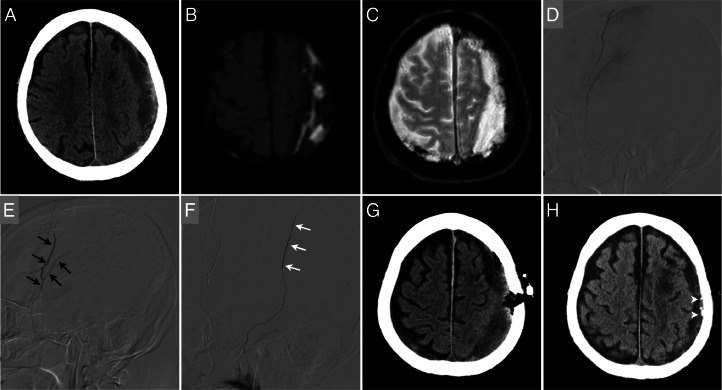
A 76-year-old male with right hemiplegia and thrombocytopenia was admitted. (A) A head computed tomography (CT) scan on admission showed a left subdural hematoma with a tough membrane and a slight midline shift. (B, C) Diffusion-weighted images and T2-star weighted images indicated the solid membrane of an organized chronic subdural hematoma. (D) A lateral view of an angiogram of the left middle meningeal artery showed the feeding arteries of the hematoma. (E, F) The left middle meningeal artery embolization was performed with heated 20% n-butyl-2-cyanoacrylate (NBCA) for the frontal branch (black arrows) and parietal branch (white arrows), followed by burr hole surgery after platelet transfusion. (G) A postoperative CT scan showed partial removal of the subdural hematoma via a burr hole. (H) After medication for immune thrombocytopenia, the hematoma resolved, and the injected NBCA was in the scar of the outer membrane (arrowhead) in 3 months.
